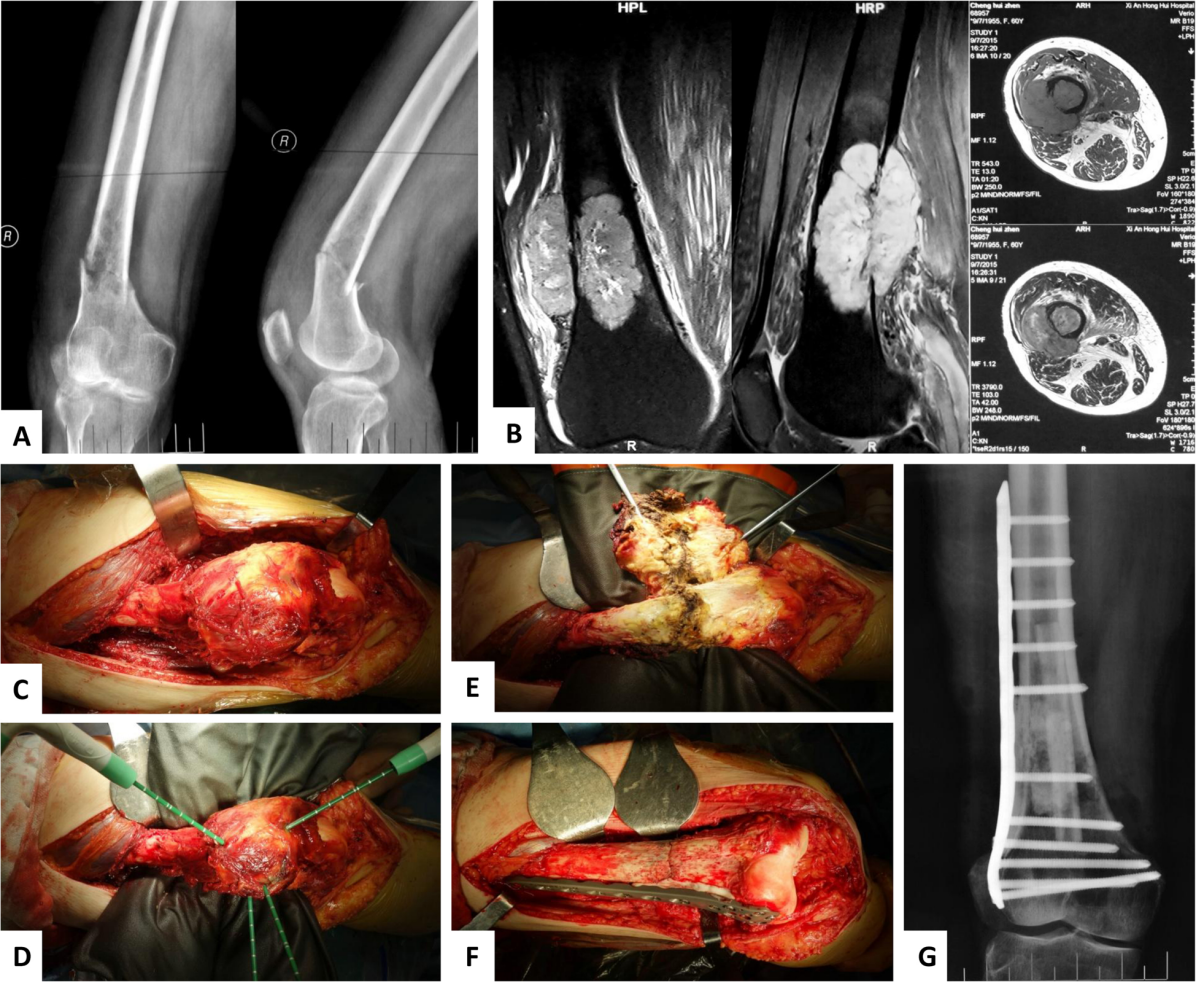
Typical procedure for pathological fracture from malignant bone tumors. a Image data of A-P and lateral X ray films showed a metastatic neoplasm from primary renal carcinoma to distal femoral diaphysis before surgery. There was visible bone destruction at the displaced fracture ends. b Preoperative MRI imaging film demonstrated the soft tumor mass and hematoma surrounding the metastatic bone lesion. c Dissect the tumor-bearing bone from surrounding normal tissues with safe margin without piercing the hematoma around the displaced fracture ends. To make sure the hematoma was not broken, the vastus intermedius was retained in situ and would experience microwave ablation process together with the lesion, soft tumor mass, and hematoma. d The microwave generator and antenna were inserted into the tumor bulk and began to deliver electromagnetic energy into the tumor bulk. e Soft tumor mass was removed and fracture ends were exposed. f The mixture materials of autologous fibular graft with bone cement were used for filling defect space and the fracture was reduced and fixed through rigid steel plate. g Postoperative A-P X ray film showed the perfect reduction and reliable fixation
